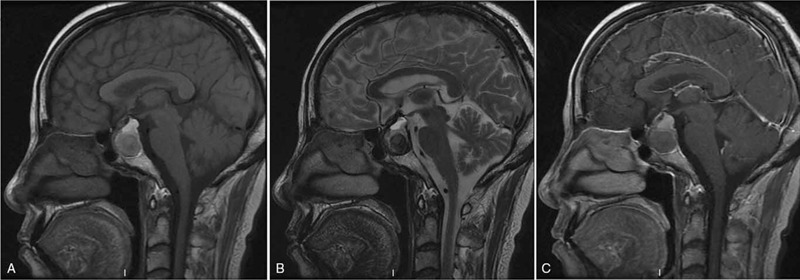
Preoperative MRI showed a large well-defined, vase-like, heterogeneous mass in the sellar region (A–C). The sign of signal stratification was found within the mass (A–C). The upper part of the mass was hyperintense, and the lower part of the mass was hypointense on both T1WI (A) and T2WI (B). The mass showed no enhancement following the intravenous administration of gadolinium (C). MRI = magnetic resonance imaging, T1WI = T1-weighted images, T2WI = T2-weighted images.
